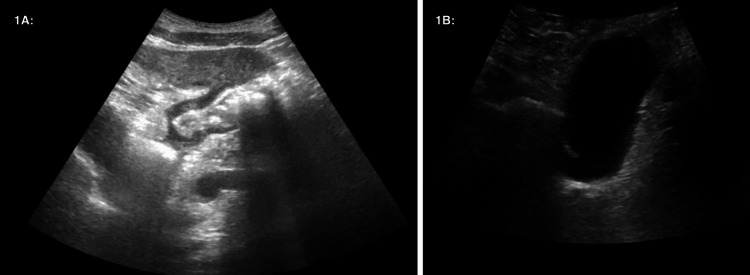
Right Upper Quadrant Ultrasound Performed at Hospital Admission. *1A:* There is mild hepatomegaly with heterogeneous poorly delineated infiltrating mass-like regions throughout the right hepatic lobe and a discrete 1.6 cm hypoechoic nodule in the left hepatic lobe. There is a discrete 1.6 cm hyperechoic nodule in the right hepatic lobe. Underlying neoplastic disease suspected. Recommend multiphase CT of the liver for further evaluation. *1B: *The distended gallbladder is sonolucent, with no gallbladder wall thickening, pericholecystic fluid, or stones. The common bile duct measures 4.8 mm.
